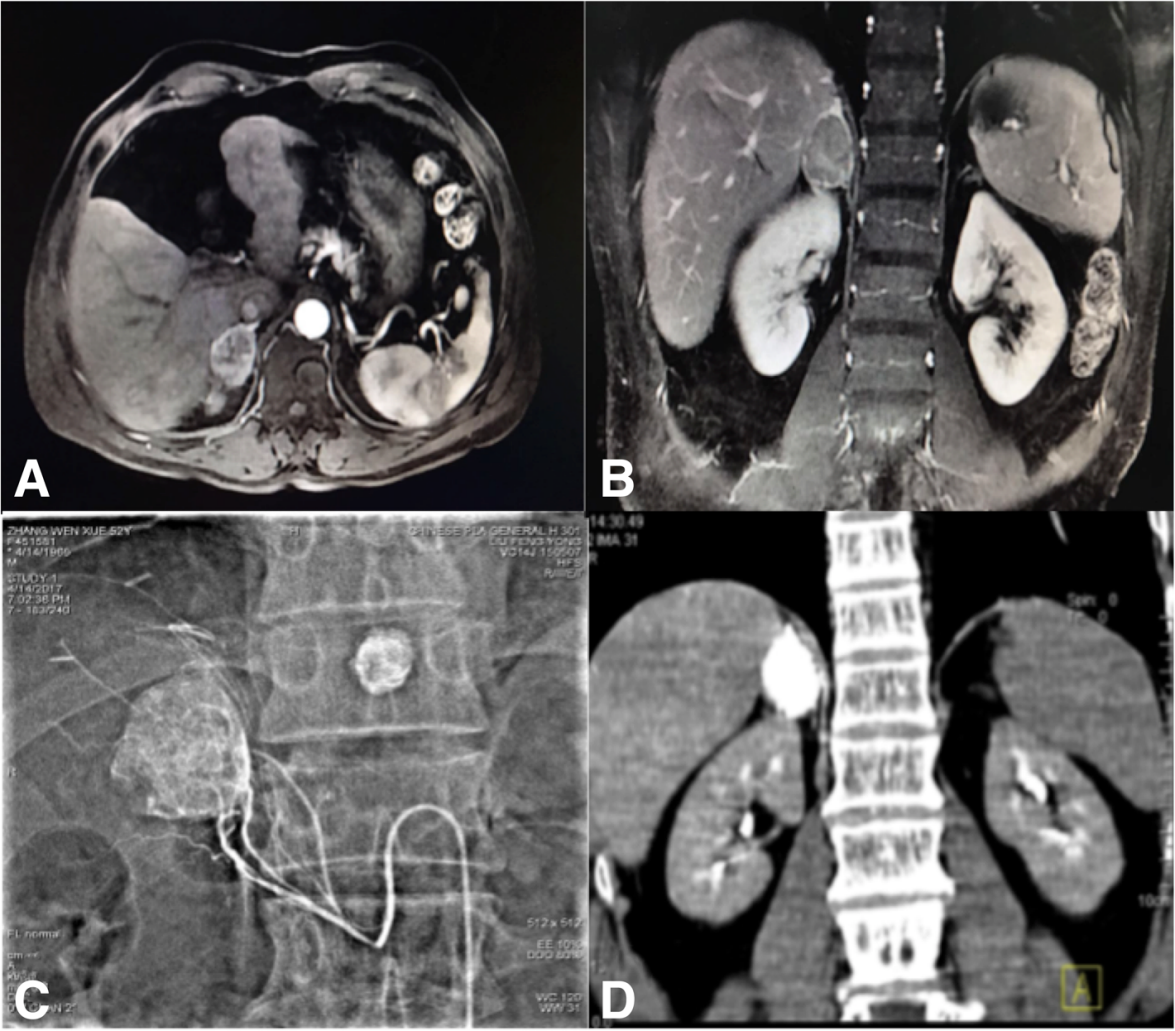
A 65-year old female patient with right adrenal metastasis of a primary liver tumor who received TACE alone. a & b Abdominal enhanced MRI, showing uneven enhancement in the arterial phase, and continuous enhancement in the venous phase. c TACE of the adrenal metastasis. d Abdominal CT at 1 month after treatment, showing favorable lipiodol deposition
